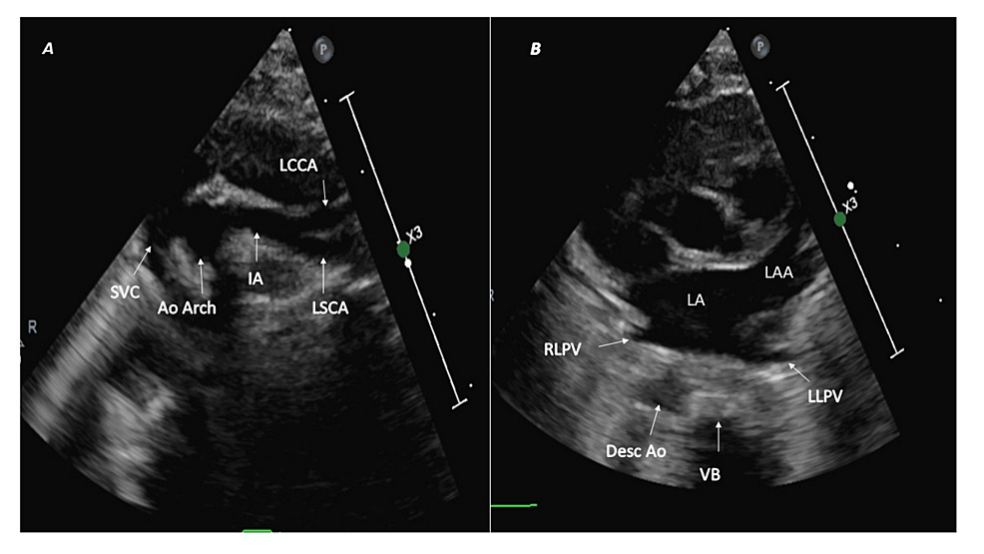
Right aortic arch with mirror branching pattern and right-sided descending aorta (A) Suprasternal short-axis view: first branch (IA) courses toward the left and bifurcates. (B) High parasternal short-axis image: right-sided descending aorta (Desc Ao) located posterior to right lower pulmonary vein (RLPV) on the right side of the vertebral body (VB). Ao Arch: aortic arch; IA: innominate artery; LSCA: left subclavian artery; LCCA: left common carotid artery; LLPV: left lower pulmonary vein; LA: left atrium; LAA: left atrial appendage.
